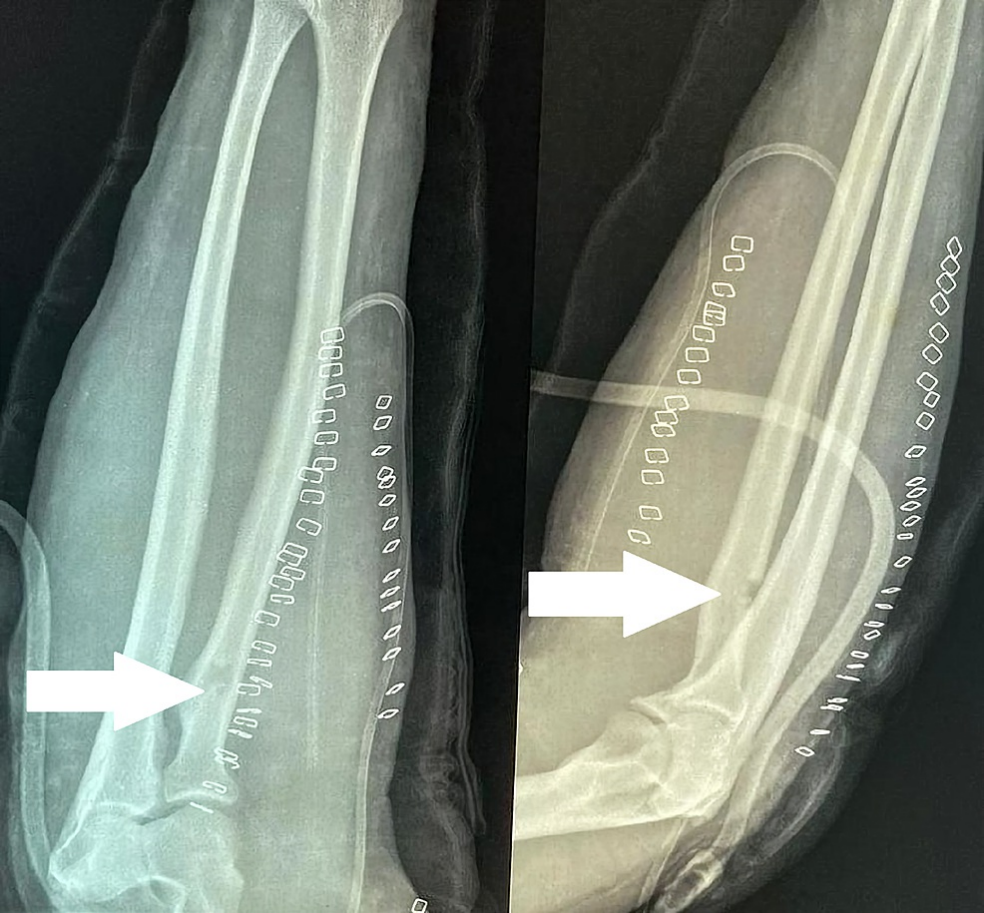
Immediate postoperative X-ray of the right forearm showing cortical breach (arrows) in proximal radius where the curettage was done
